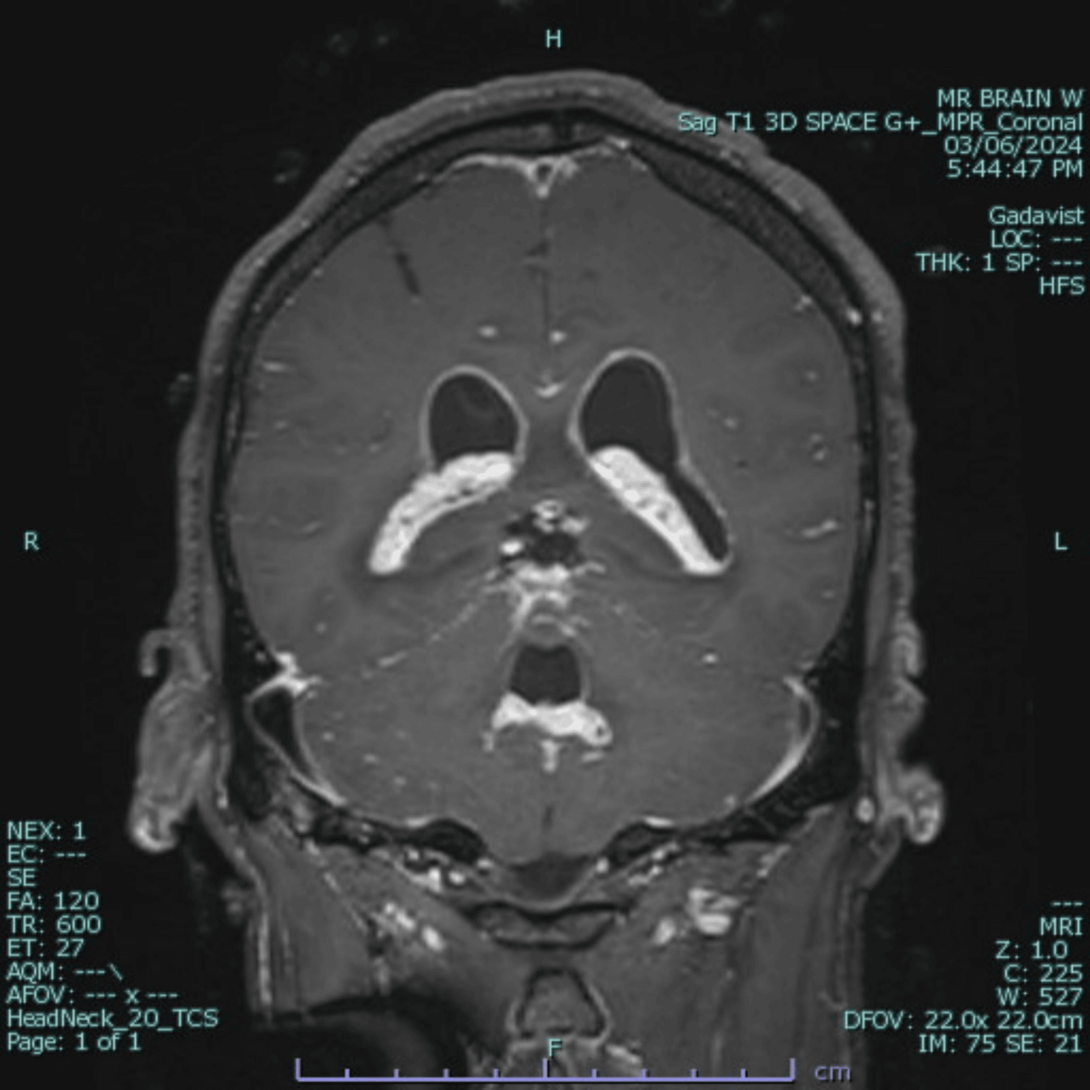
Coronal T1-weighted post-contrast MRI showing ventriculomegaly, leptomeningeal enhancement of the pia mater and choroid plexus extending to the foramina of Magendie and Luschka, and edema of the cerebellar dentate nucleus
